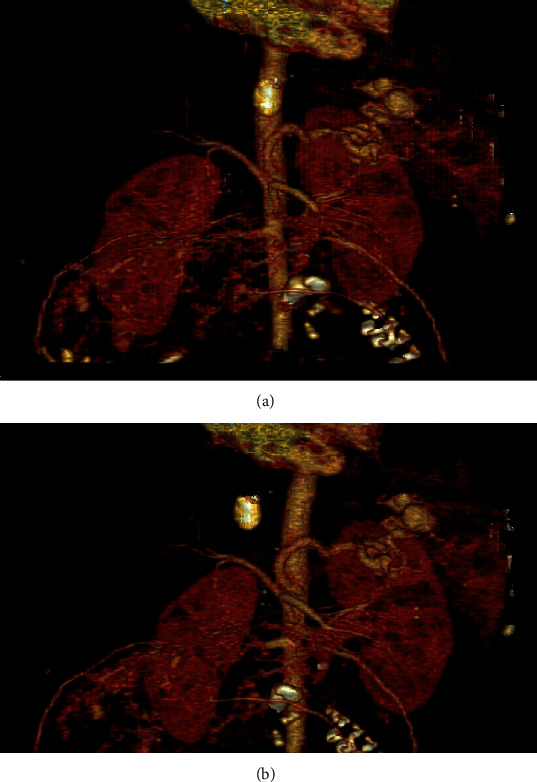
(a) CT angiogram 3-D reconstruction showing the tortuous splenic artery aneurysm (anterior view). (b) CT angiogram 3-D reconstruction showing the tortuous splenic artery aneurysm (posterior view).
